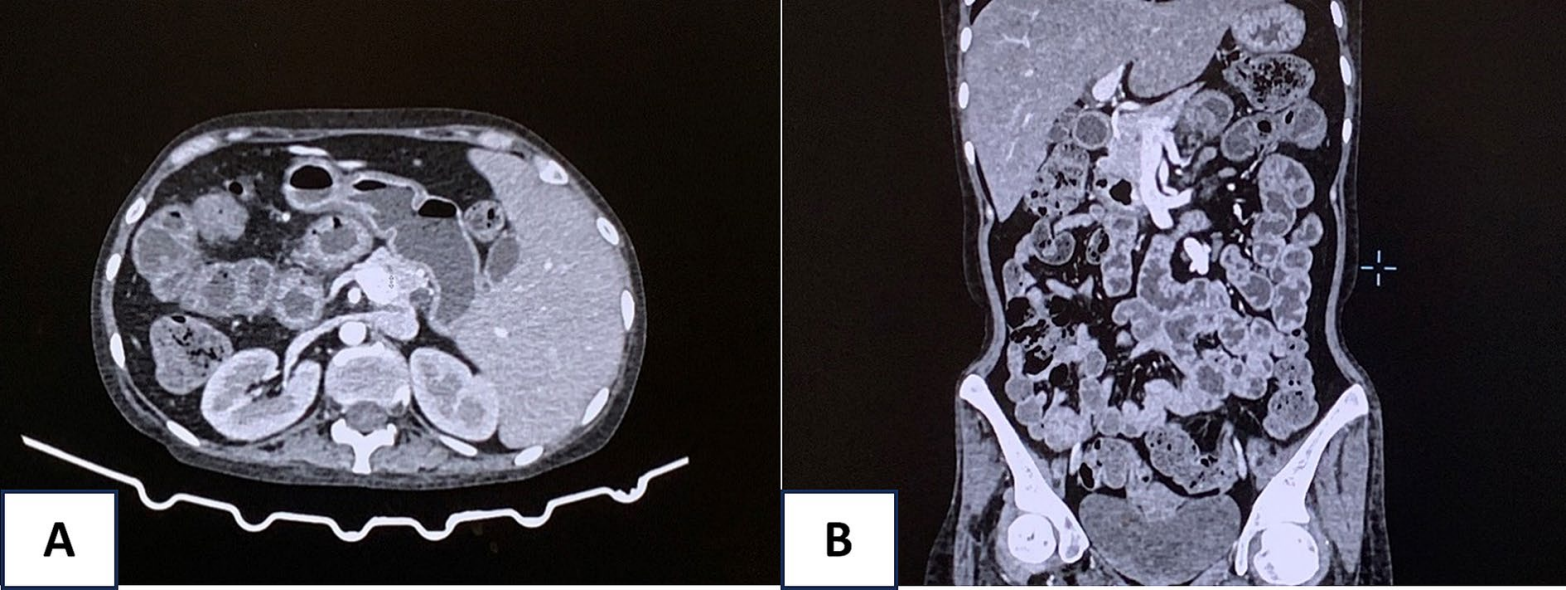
Abdominal computed tomography (CT) scan revealed edema and a thickening wall throughout the intestinal loops from the duodenum downward (A, axial view and B, coronal view).
